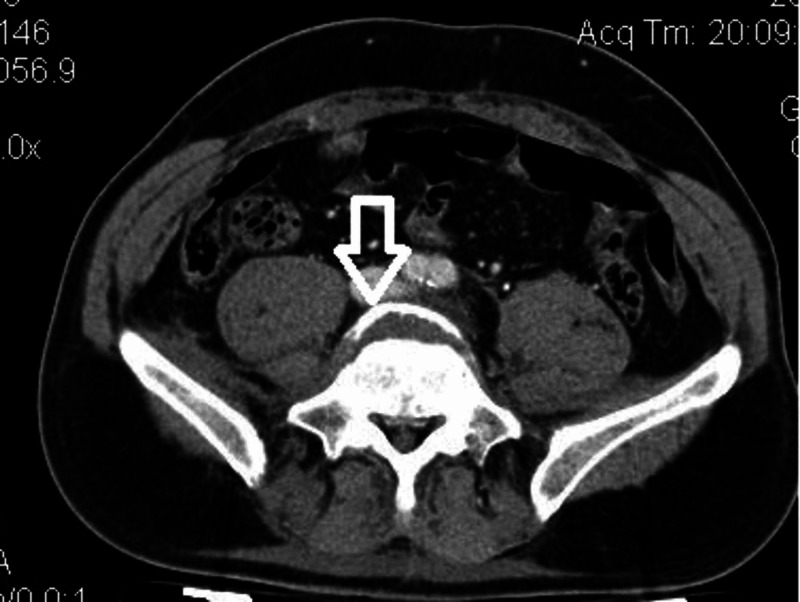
Preoperative axial CT scan at the segment L4-L5 showing double contour (white arrow), indication of olisthesis.
